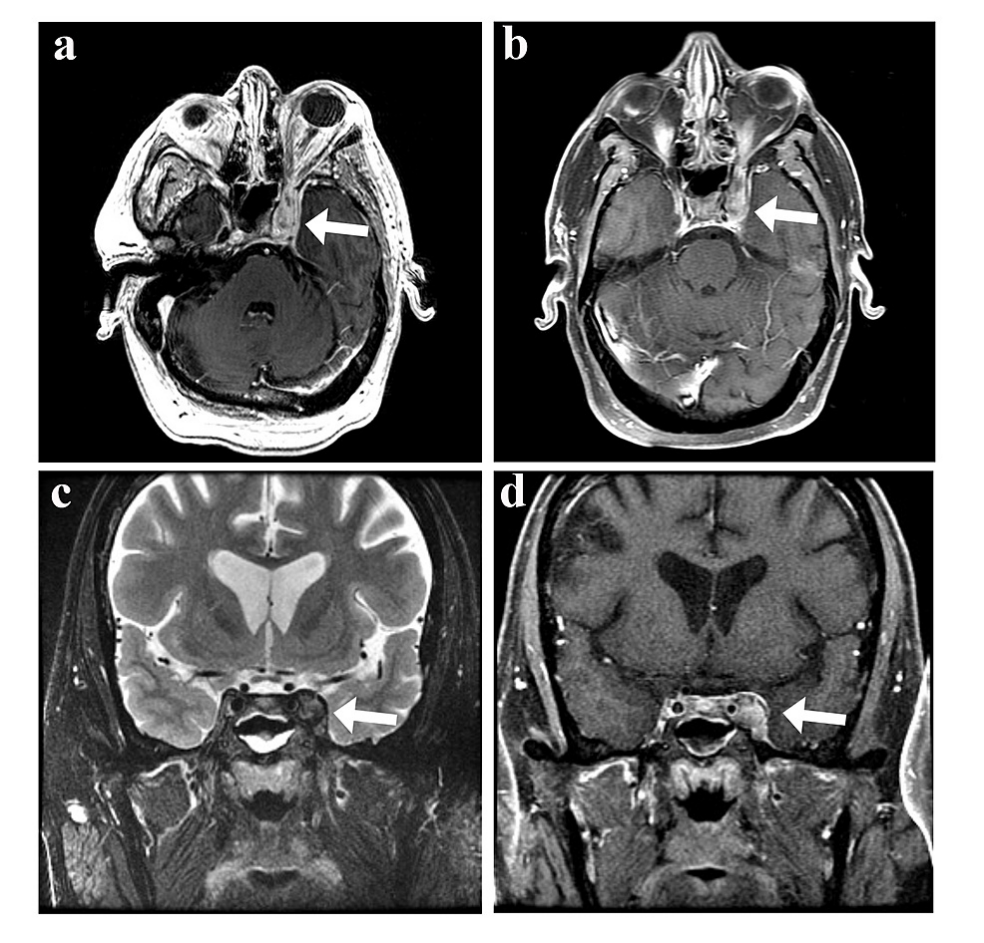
MRI of an inflammatory left cavernous sinus process consistent with Tolosa-Hunt syndrome T2 axial FLAIR (a) and FSE coronal (c) images showing bulky perineural tissue extending into the left cavernous sinus. The perineural tissue has heterogeneous post-contrast enhancement and slightly decreased enhancement centrally consistent with a component of thrombosis on post-contrast T1 axial (b) and coronal (d) images MRI: magnetic resonance imaging; FLAIR: fluid-attenuated inversion recovery; FSE: fast spin-echo
